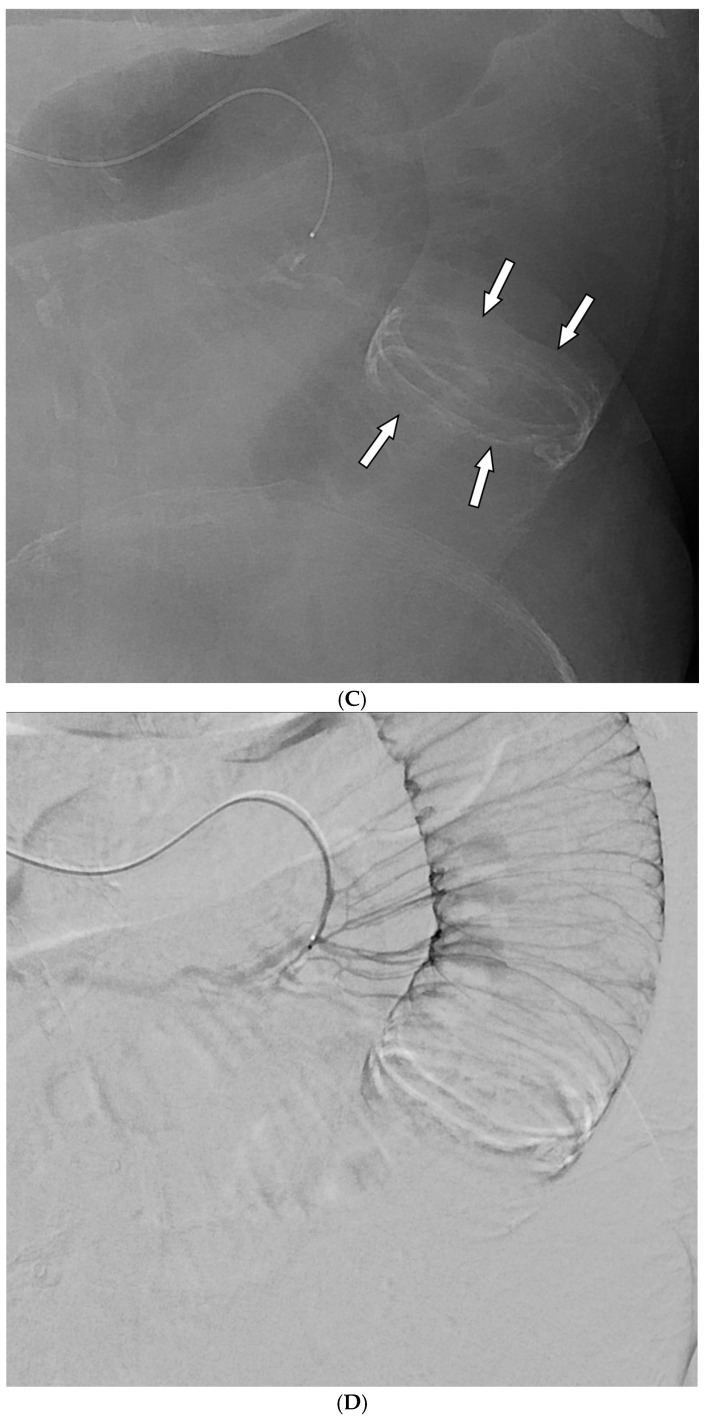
A 67-year-old woman presented with hematochezia. (A) Contrast-enhanced coronal CT image shows luminal contrast extravasation (arrow) at the jejunal loop. (B) Selective jejunal arteriography shows contrast extravasation (arrow). (C) Several levels of the vasa recta (arrows) were embolized with quick-soluble gelatin sponge particles (150–350 µm). (D) Post-embolization arteriography shows devascularization of the previously embolized vasa recta. No further bleeding occurred before the patient’s death one month after embolization.
